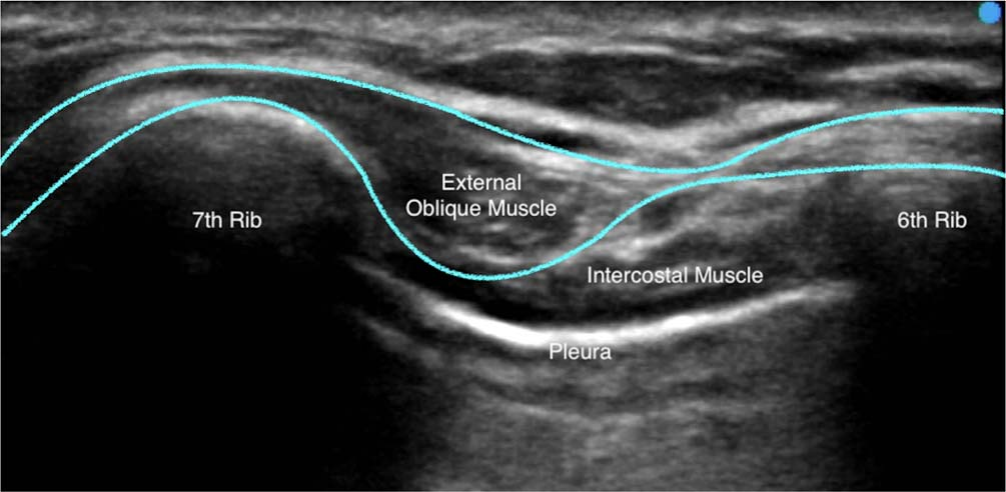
A typical ultrasound image obtained while administering the EOI block. The external oblique muscle, intercostal muscles, sixth rib, seventh rib, and pleura is labelled. The border external oblique muscle border is outlined.
